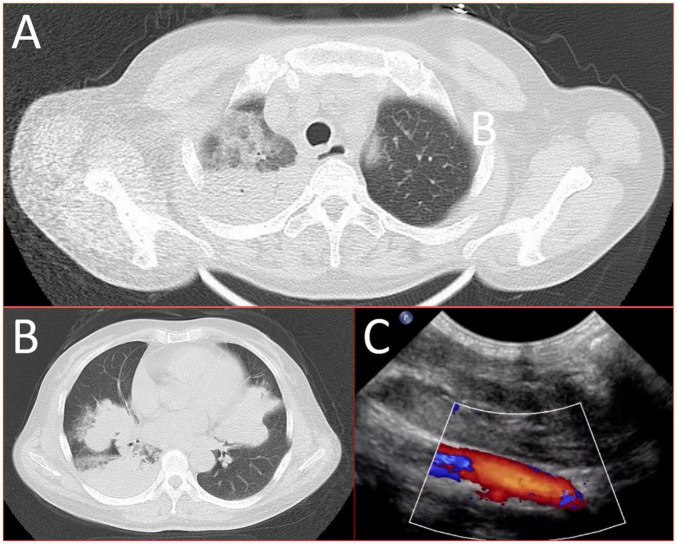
16-slice computerized tomography images of the chest in the exacerbation stage of the patient. (A) Upper lobe; (B) lower lobe and ultrasound images of the left internal jugular vein at the exacerbation stage. (C) The continuity of the external wall of the left internal jugular vein root was interrupted, the internal diameter of the left internal jugular vein root was approximately 3.2 mm, and the internal diameter of the upper segment was approximately 7.0 mm. The sound transmission in the middle and lower segments of the vein was poor, and faint echo filling was visible).
